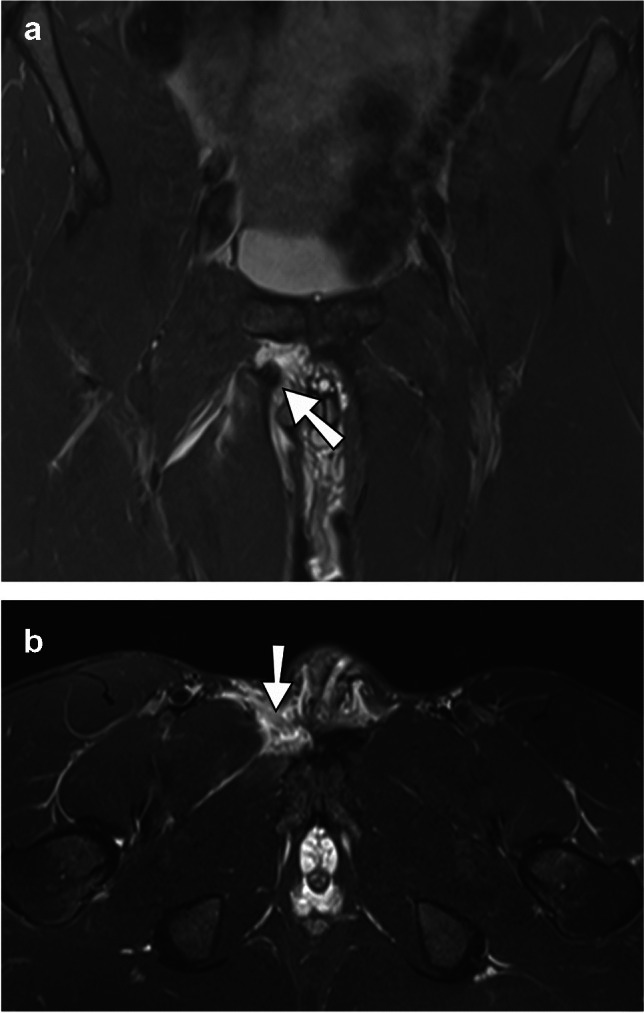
Complete tendon tear. Coronal (a) and axial (b) T2-weighted and fat-suppressed MR images of the groin region. Complete tear of the right proximal adductor longus tendon at the pubic ramus (arrow) with distal retraction, loss of muscle tension, and surrounding edema
